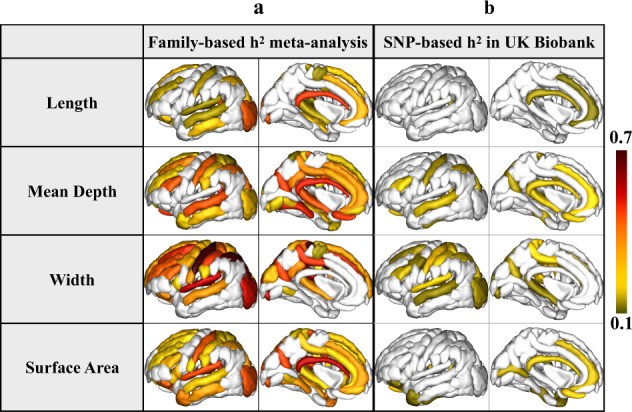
Heritability estimates. Heritability estimates (*h*^2^) are mapped, for each bilaterally averaged sulcal descriptor. a The results of the inverse-variance weighted meta-analysis of the heritability estimates across three family-based cohorts QTIM, HCP, and GOBS highlight an overall heritability profile across 3030 individuals. b Heritability estimates (*h*^2^) calculated from sulcal features extracted from MRI scans of 10,083 unrelated individuals scanned as part of the UK Biobank were calculated using the genome-wide complex trait analysis (GCTA) package. The regional sulcal metrics that were found to be significantly heritable in the large population sample largely overlap with those found to be most highly heritable across the family-based studies. We highlight only regions that had significant heritability estimates in sulci that had an ICC > 0.75 (see Supplementary Data 2–4 for sulcal-based values of ICC). Significant regions survived Bonferroni correction for multiple comparisons across all bilateral traits and regions (*p* < 0.05/(61 × 4)); darker red colors indicate higher heritability estimates. The left hemisphere was used for visualization purposes.
